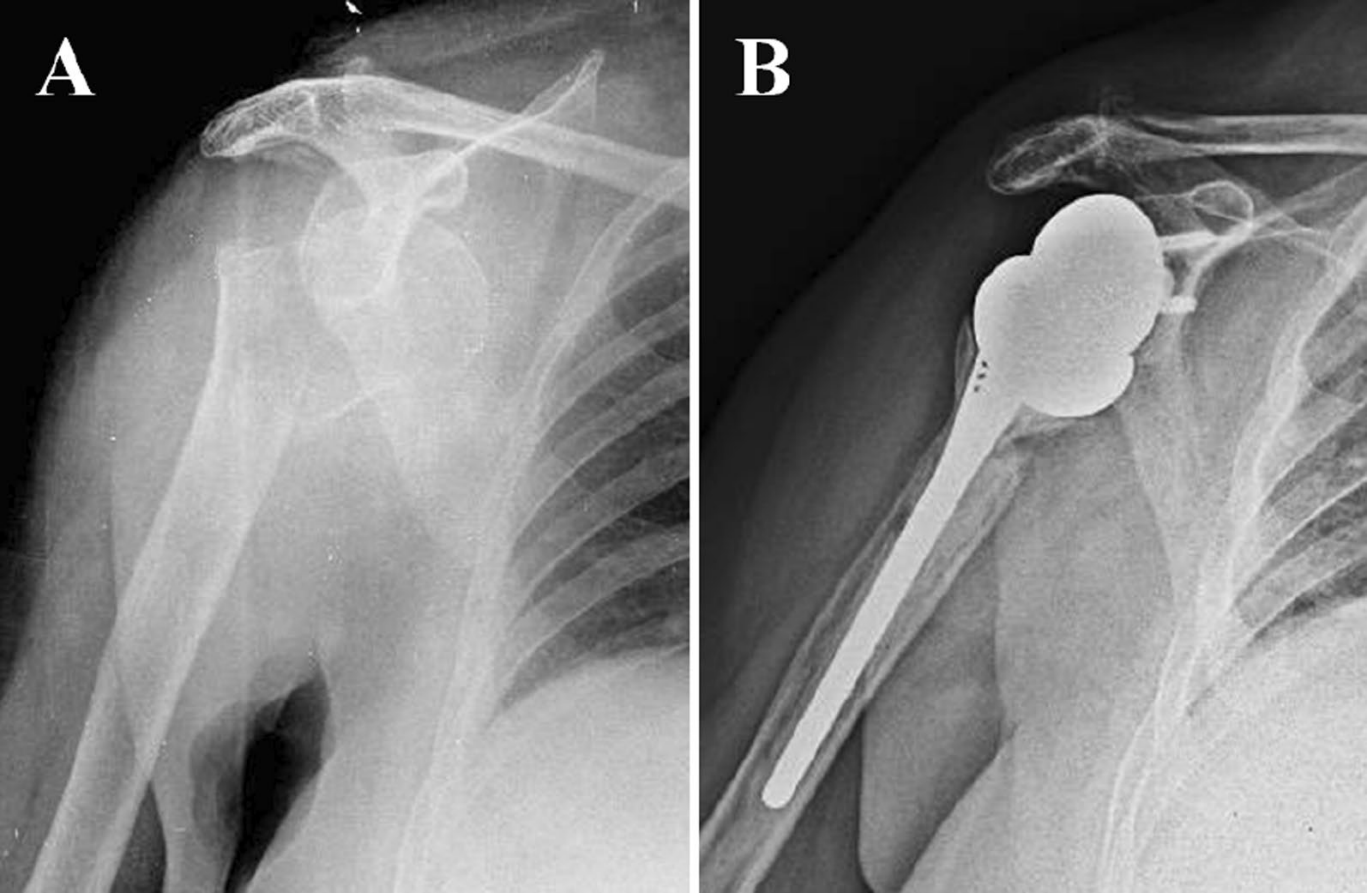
These images show X-rays of the same patient, with a complex dislocated fracture of the proximal humerus (A) and his follow-up after 7 years (B)
